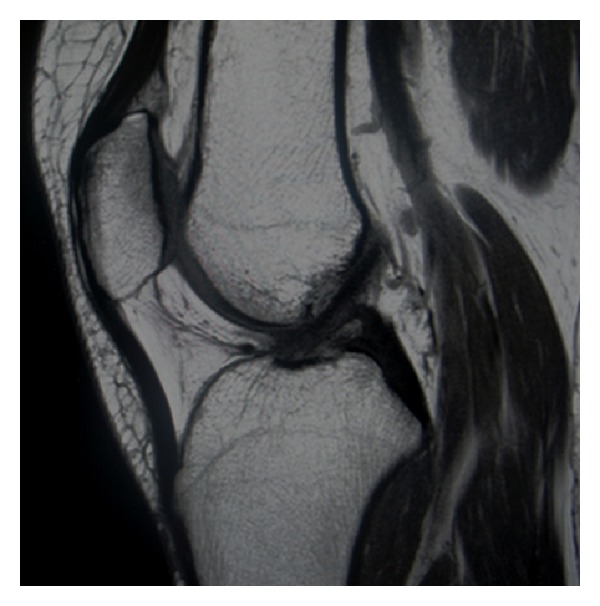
MRI images of patient at final follow-up showing absence of any ganglion cyst.
